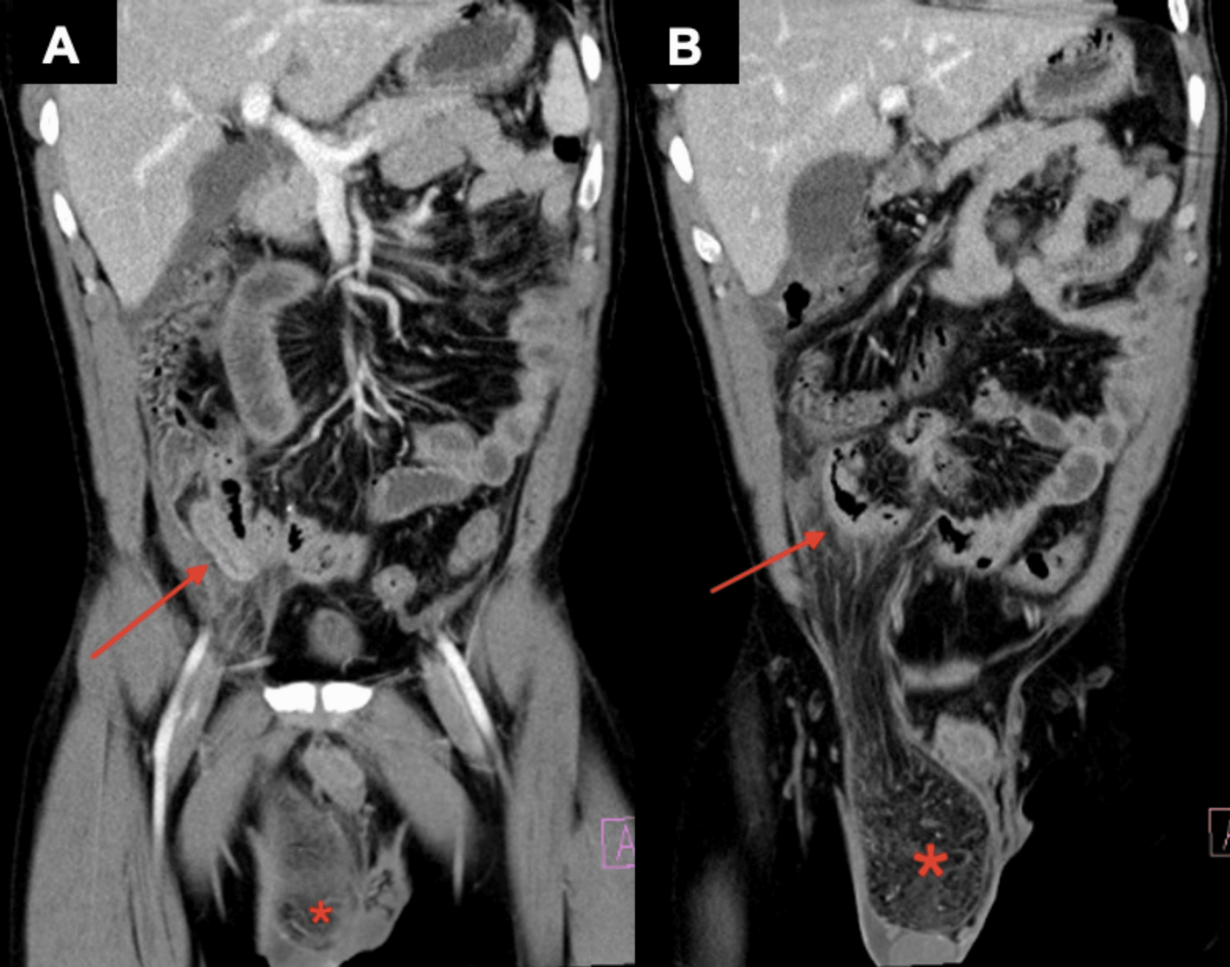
Coronal (A and B) CT images of the abdomen and pelvis with intravenous contrast demonstrating a right-sided Amyand's hernia with perforated appendicitis There is radiographic evidence of an inflamed appendix (red arrow) protruding into the hernia sac (red asterisk). CT: computed tomography
